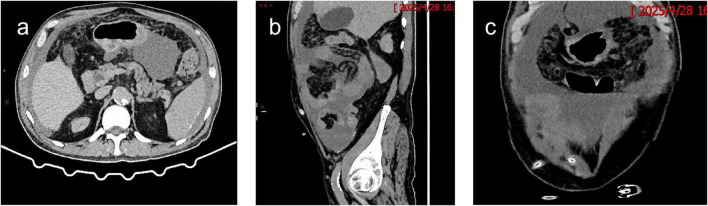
CT of the abdomen on the axial plane (a), the sagittal plane (b), and the coronal plane (c).
